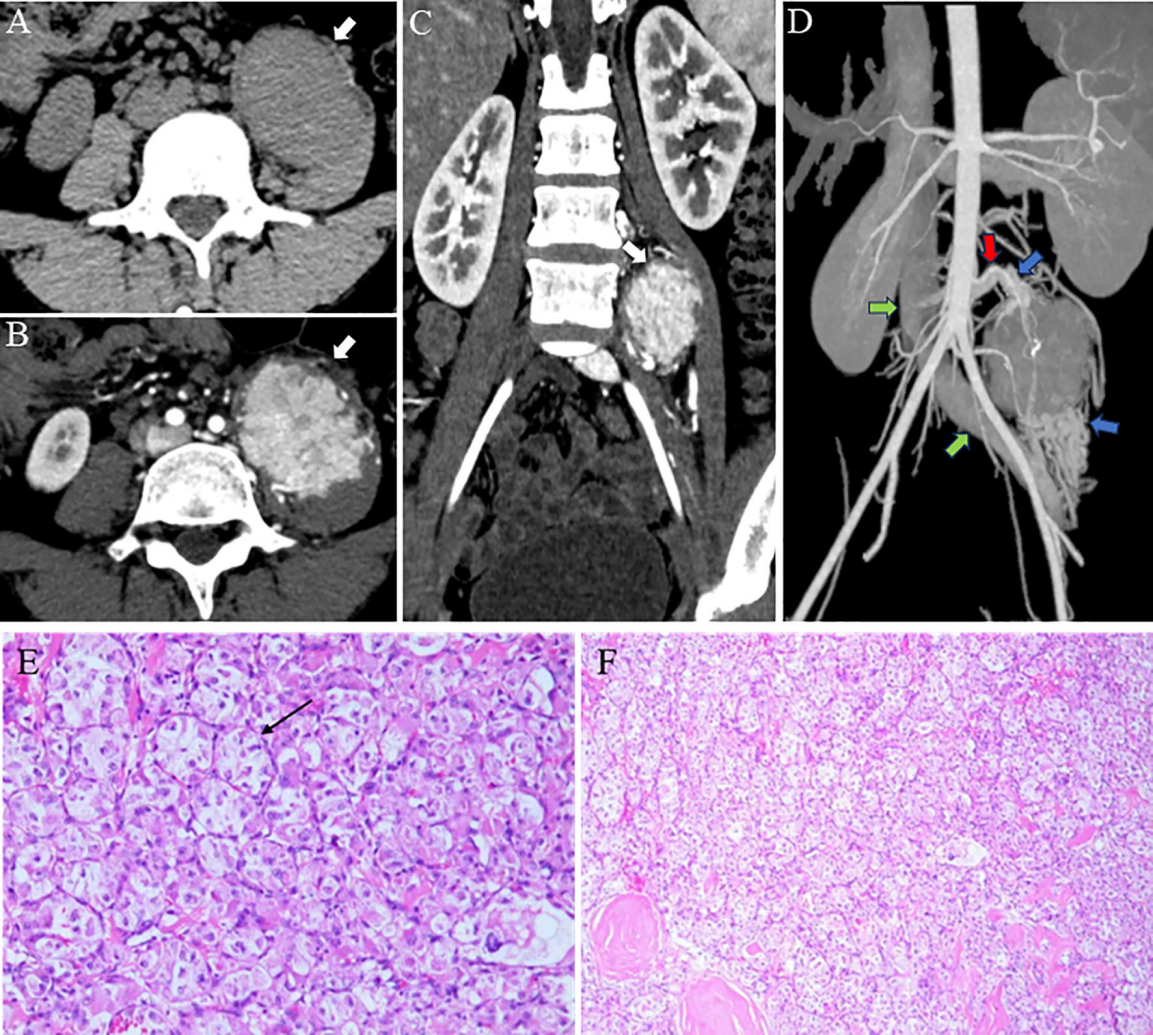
Imaging findings. (A) A clearly defined, isodensed mass located within the left retroperitoneal psoas major muscle is revealed in CT. (B) A highly vascular tumor with internal nodularity and lobulated margins (white arrow) is seen on a contrast-enhanced CT axial image. (C) A reconstructed coronal image displaying the “split-fat” -a radiological term describing fusiform fat-density shadows encircling the tumor’s upper and lower poles, sharply contrasting with its soft-tissue density, reflecting fat displacement caused by tumor expansion (white arrow). (D) A maximum intensity projection image showing large peripheral vessels located at the superior and inferior regions of the tumor, revealing multiple branch supply arteries originating from the abdominal aorta (red arrow), along with tortuous and thickened veins surrounding the mass (blue arrow), which ultimately drains into the inferior vena cava (green arrow). (E, F) Tumor cells are organized in an alveolus-like structure, with delicate blood sinuses present in the interstitium (black arrow).
